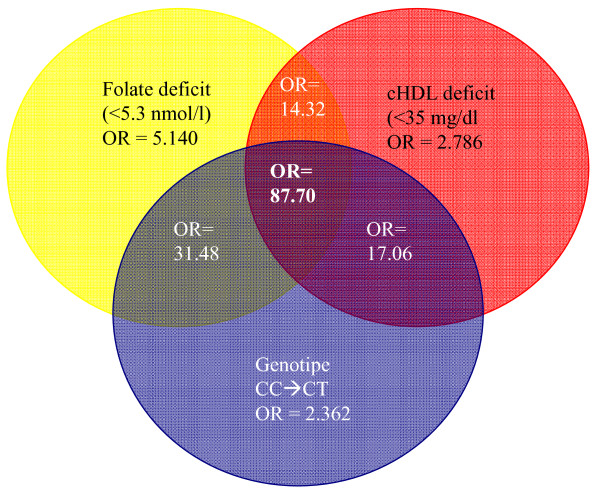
Cumulative ORs: Folate, cHDL and MTHFR C677T genotype. Cumulative effect of risk factors in homocystein prevalence. HDL: High Density Lipoproteins. MTHFR: methylenetetrahydrofolate reductase. C: Cytosine; T: Thymine; CC: normal genotype; CT: partial mutation; TT: mutation in homozygosis.
